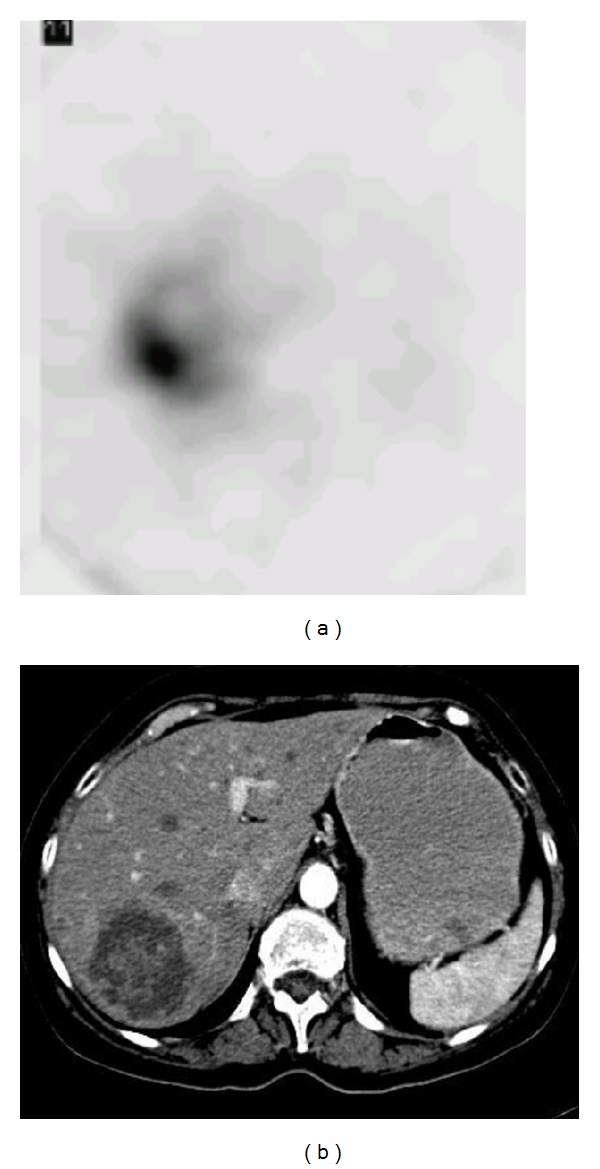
(a) Enlarged necrotic tissue secondary to previous chemoembolization (nonradioactive lipiodol) and (b) correlated CT slice.
